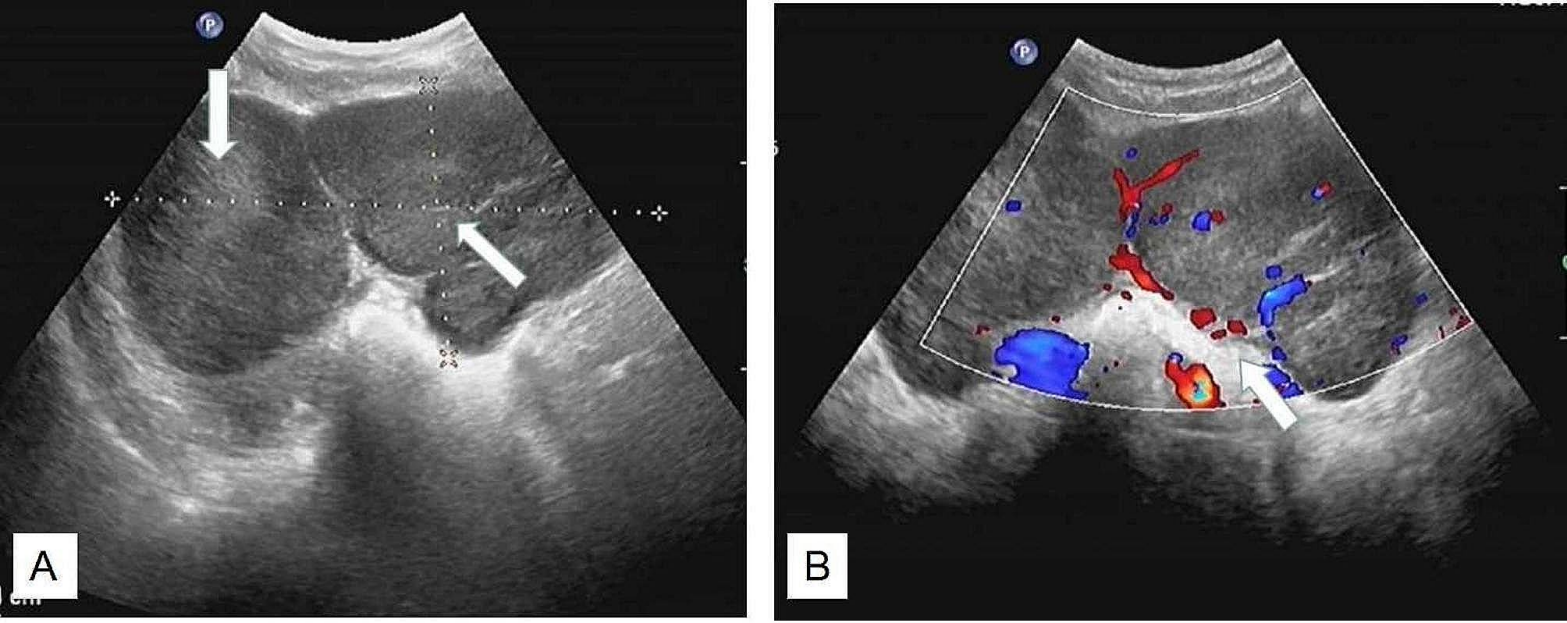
Ultrasound images: (A) Ultrasonic probing there are substantial uneven low echo masses sized of 112 mm*58 mm*98 mm (left ovary) and 144 mm*73 mm*83 mm (right ovary) in the pelvic cavity, cling to the uterine corpus, the boundary of the masses are relatively clear. (B) the color Doppler shows blood flow signal around the masses (Case 7)
